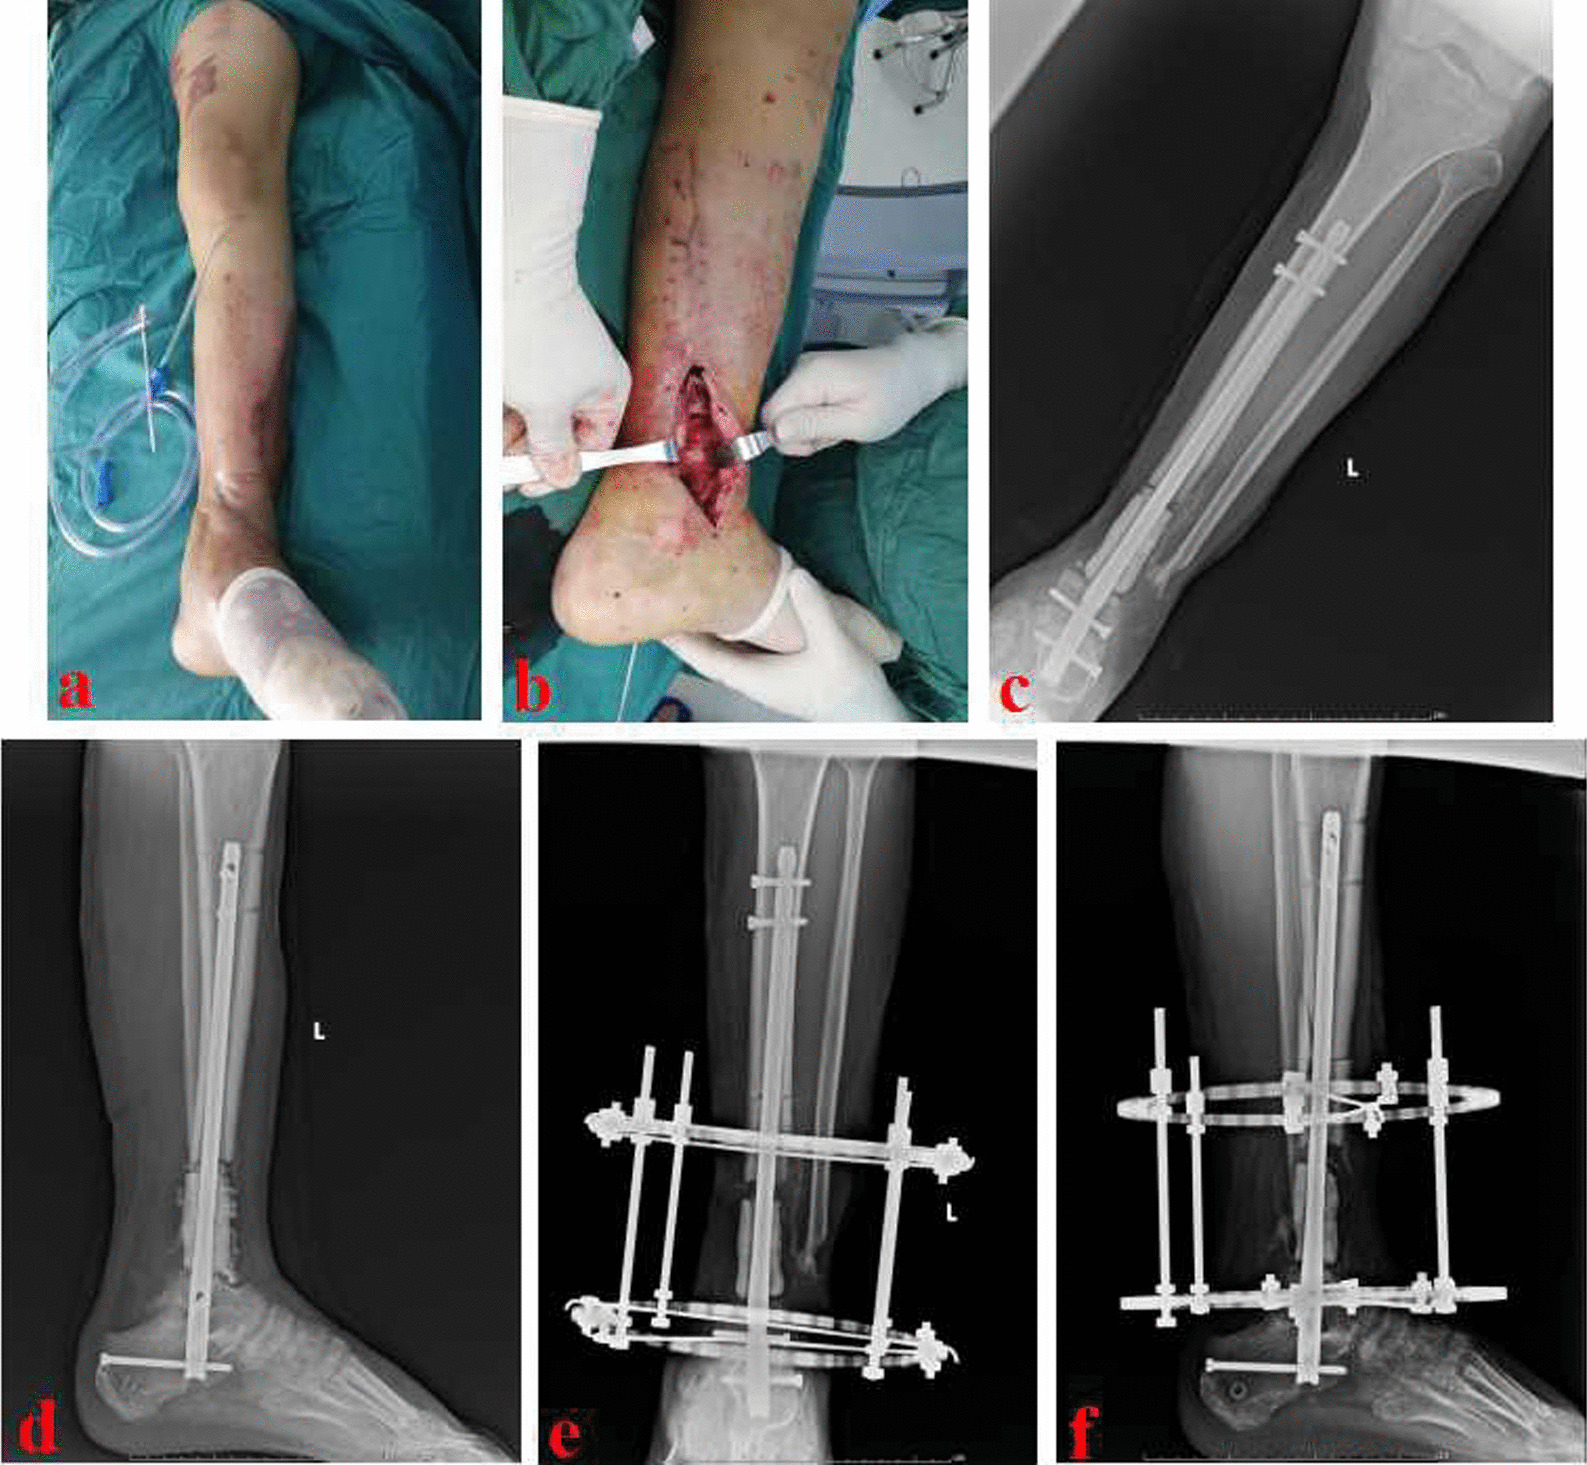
The patient underwent bone reconstruction through the MHT technique. a Appearance of the lower leg after wound healing; b After removing the bone cement, obvious bone defects (about 8.0 cm) was observed in the wounds; c and d X-ray films after the insertion of a retrograde tibiotalocalcaneal arthrodesis nail and antibiotic-loaded calcium sulfate; e and f After two weeks, the annular bone transport frame was installed. MHT: modified hybrid transport
